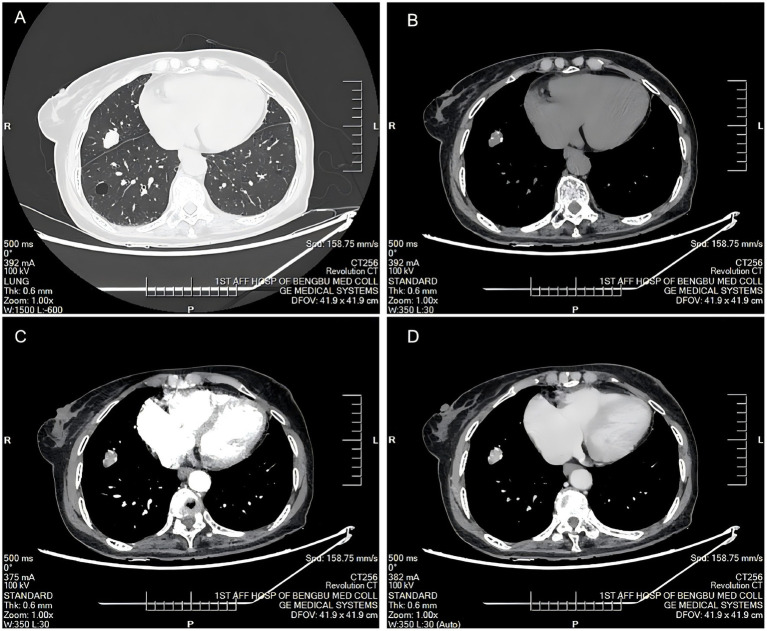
Irregular solid nodule of pulmonary sclerosing pneumocytoma (PSP) on CT. White arrows indicate the lesion, lobulation, and pleural indentation. An irregular solid nodule is seen in the lateral segment of the right middle lobe, with lobulation, fine spiculation, and punctate calcification. (A) Non-contrast lung window. (B) Non-contrast mediastinal window. (C) Arterial phase. (D) Venous phase showing mild-to-moderate progressive heterogeneous enhancement.
